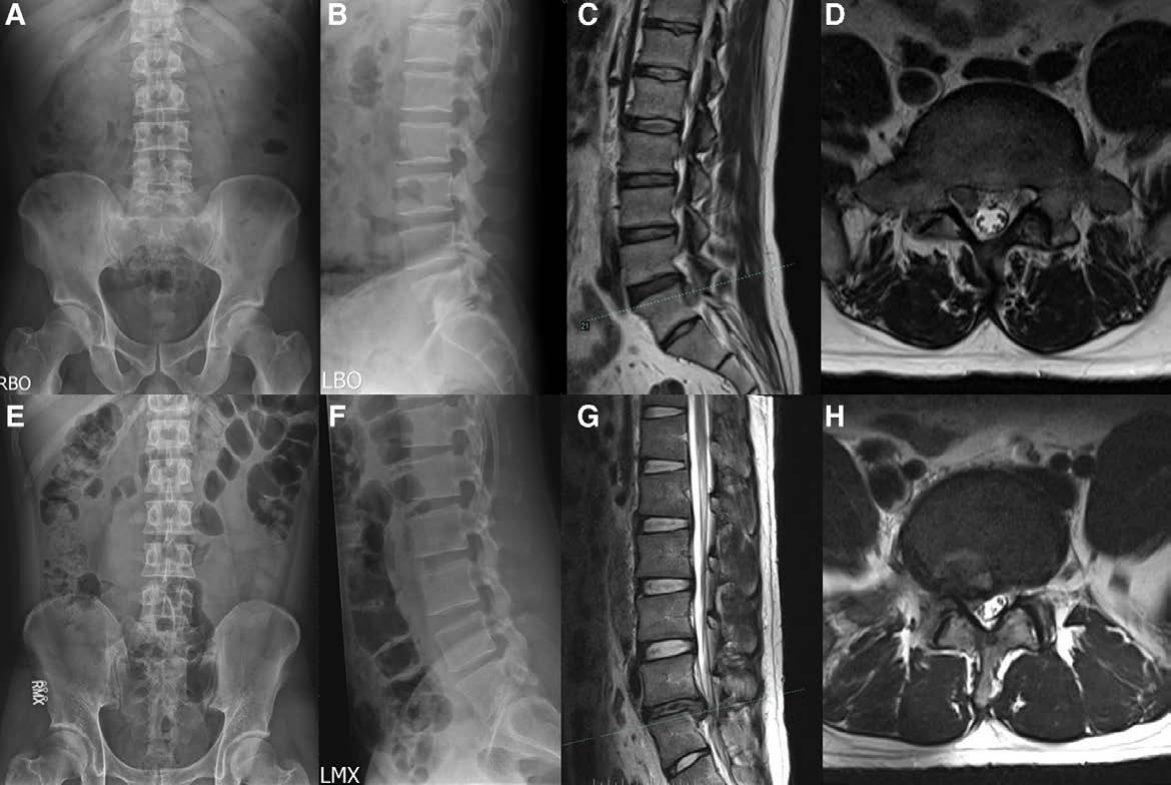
Two patients with lumbosacral transitional vertebrae. (a-d) A 36-year-old male presented with low back pain with pain radiating to the right lower limb for 3 months. (a, b) Radiographs of the lumbar spine showed L5 sacraliation. (c, d) Preoperative MRI showed L4-S1 disc herniation with downward migration of the right ruptured disc. (e-h) A 26-years-old male presented with right buttock pain radiating to the right lower limb. (e, f) Radiographs of the lumbar spine showed S1 lumbarization. (g, h) Preoperative MRI showed L5-S1 disc herniation on the right side. MRI = magnetic resonance imaging.
